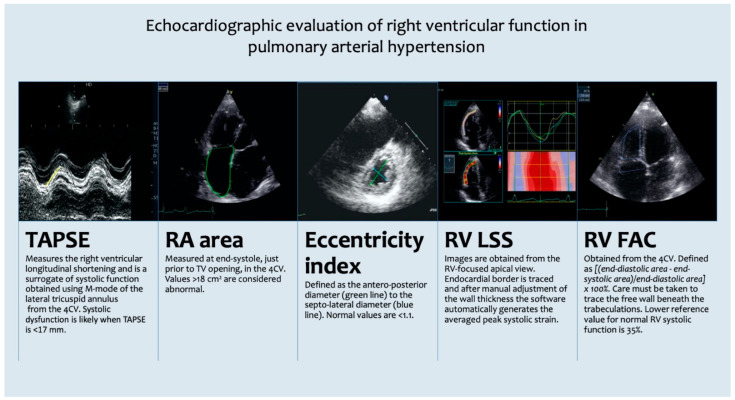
Echocardiographic evaluation of right ventricular function in pulmonary arterial hypertension. 4CV: four-chamber view; FAC: fractional area change; LSS: longitudinal free-wall strain; RA: right atrium; RV: right ventricular; TAPSE: tricuspid annular plane systolic excursion.
